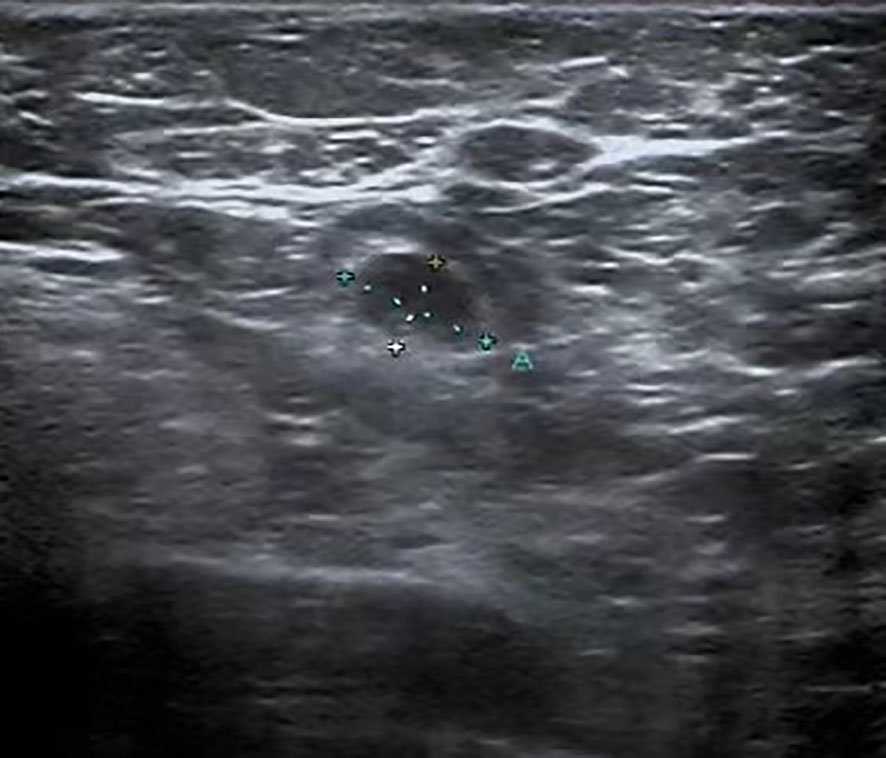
Ultrasound image of the axilla: The largest lymph node in the right axilla, measuring 9.7mm by 5.9mm, has lost its normal architecture.
